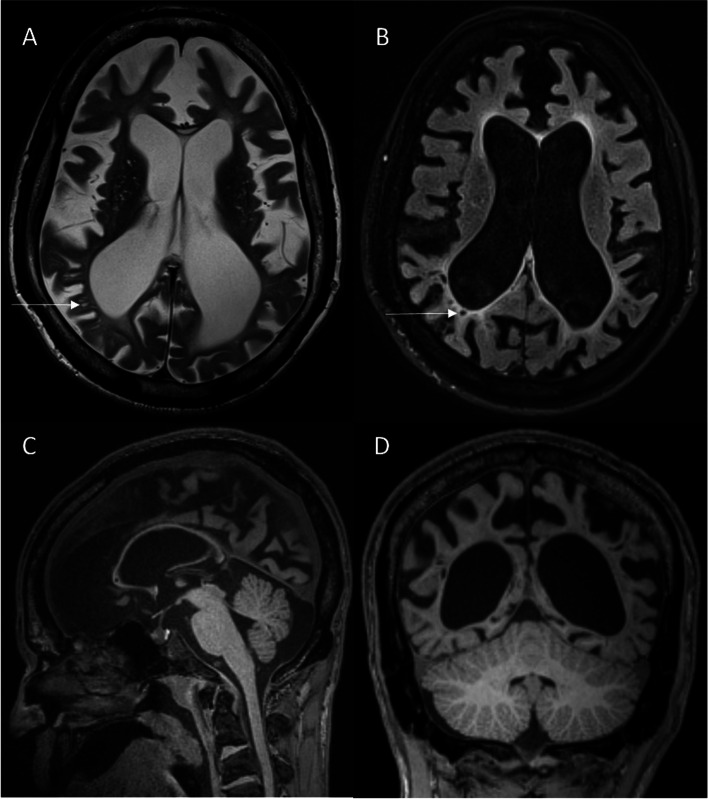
Brain MRI at the age of 28 years. Transversal T2-weighted image (A) shows ventriculomegaly and enlarged cortical sulci due to brain atrophy. There is associated enlargement of the perivascular spaces (arrows). B Fluid-attenuated inversion recovery (FLAIR) sequence shows only a slight periventricular increase in signal intensity, which can be attributed to hypothetical dysmyelination. On 3D-T1-weighted images, sagittal reconstruction (C) demonstrates a thin corpus callosum and coronal reconstruction (D) shows the convincingly reduced volume of the supratentorial hemispheres, whereas the cerebellum appears normal
